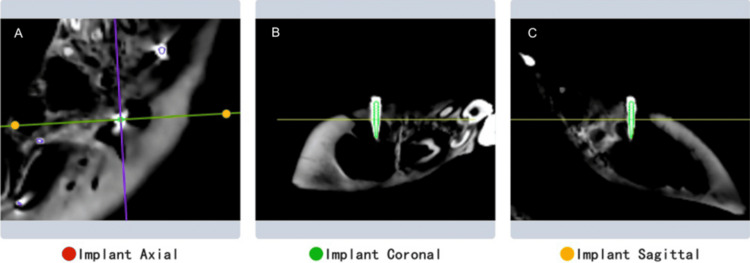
Overlay of the preoperative plan and postoperative CBCT image Visualization of the miniscrew position as planned pre-operatively (green outline) and its actual position post-operatively. Cross-sectional views showing the alignment of the planned and placed miniscrews. A: axial view; B: coronal view; and C: sagittal view CBCT: cone beam computed tomography
